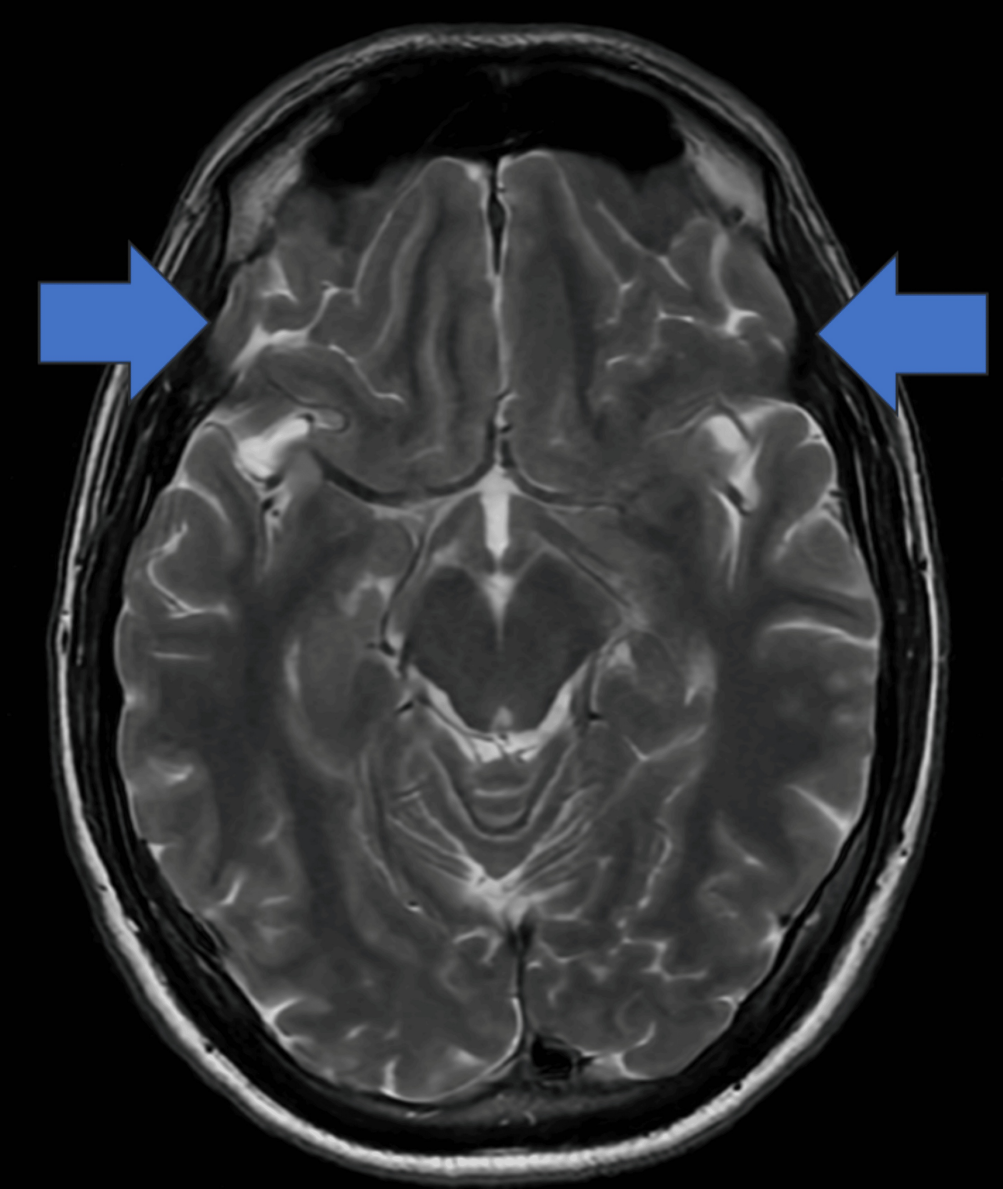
T2-weighted MRI of the brain with and without contrast (3/29/23) Impression: (1) The arrows represent the minimal increased dural and leptomeningeal enhancement in the bilateral frontotemporal regions. (2) No MR evidence for increased intracranial pressure was shown.
